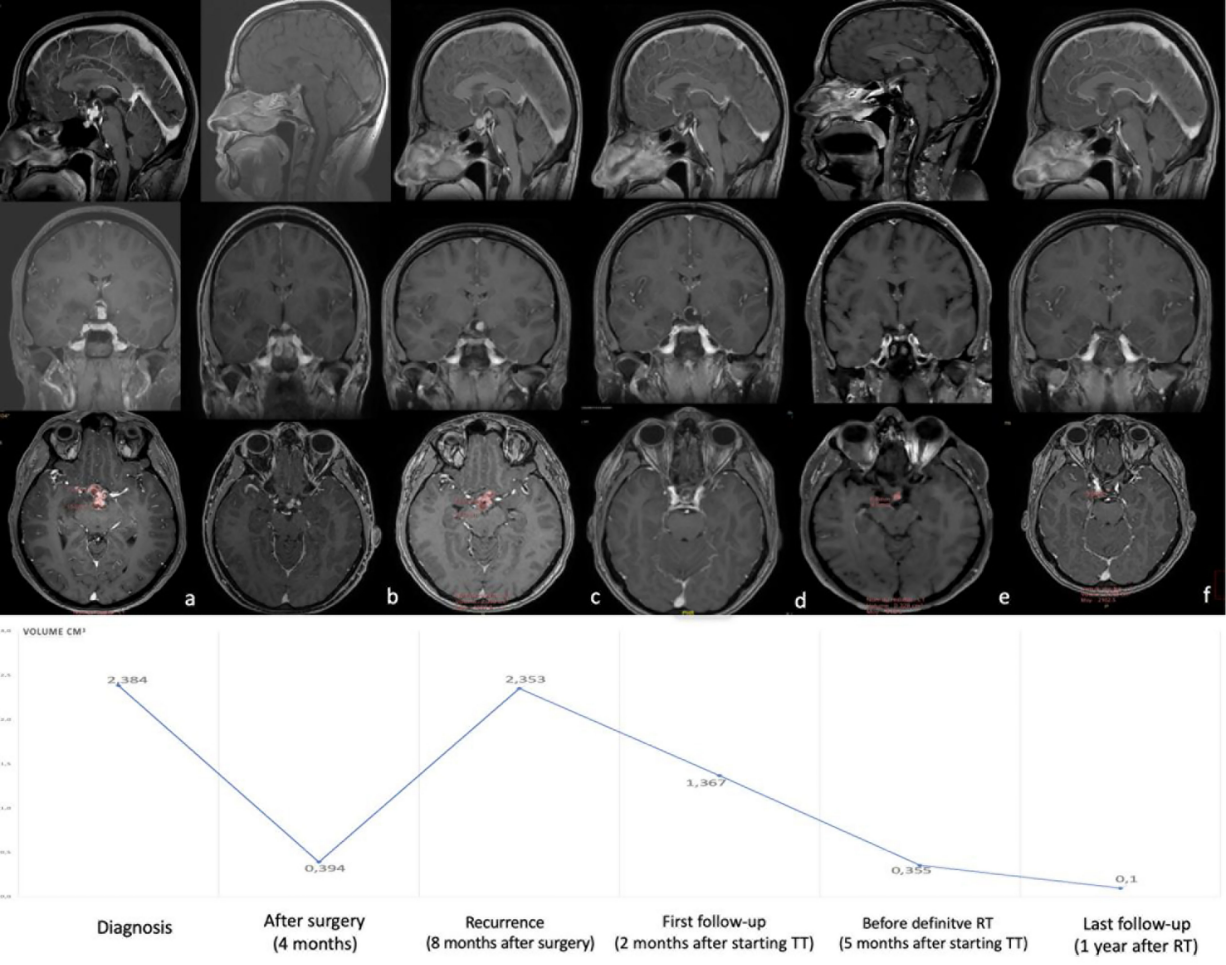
Post-gadolinium axial, coronal and sagittal T1WI MRI images, representing the clinical course in case 1. (A, B) Shows the tumor volume and presentation at time of diagnosis (25.4 x 15.0 mm maximal axis and 2.384 cm3 volume) and after surgery (maximal axis: 7.5 x 11.5 mm, volume: 0.394 cm3). (C) Shows tumor recurrence/regrowth at 12 months postoperatively (13 x 24 mm and a volume of 2.353 cm3). (D, E) Show dramatic and rapid reduction in tumor volume at 2 months (80 %) and 4 months (90%) after starting combined anti-BRAF/MEK therapy. (F) Shows results at 1 year after final radiotherapy (near complete response). Volume curve has been reported in the inferior part of figure. TT: B-RAF and MEK inhibitor targeted therapy; RT: radiotherapy.
